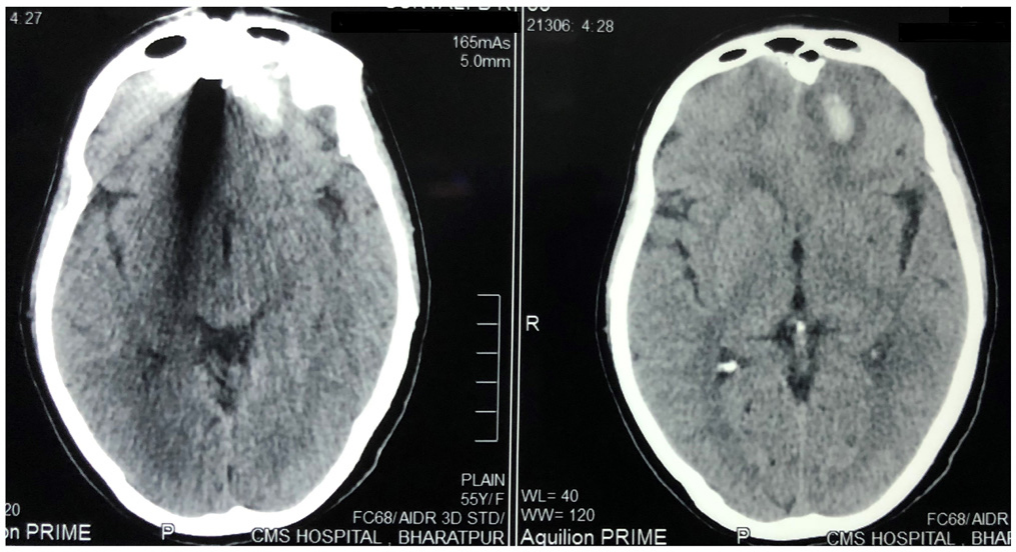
Plain Computed Tomogram of the brain showing the left frontal contusion and metal artefact due to foreign body.
